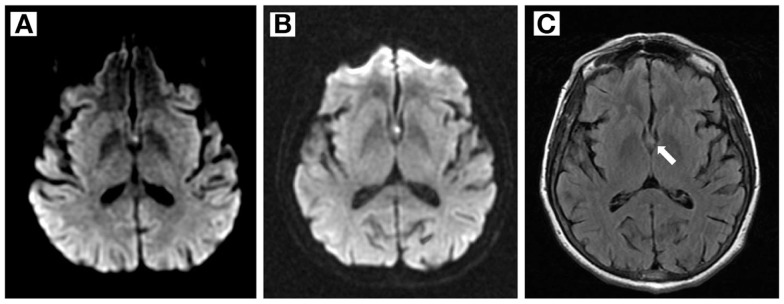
Magnetic resonance imaging findings. (A,B) Diffusion- weighted imaging shows a small hyperintense area in the left fornix 1 and 3 days after symptom onset, respectively. (C) FLAIR image 3 days after symptom onset.
